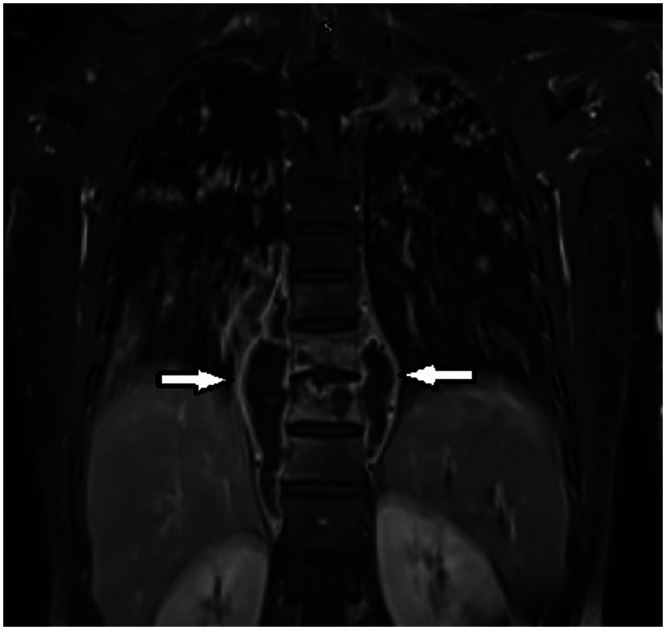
A spinal magnetic resonance imaging sagittal view showing heterogeneous enhancement of the T9, T10, and T11 vertebral body and bilateral paraspinal collection/cold abscess at the same level anteriorly (arrows).
